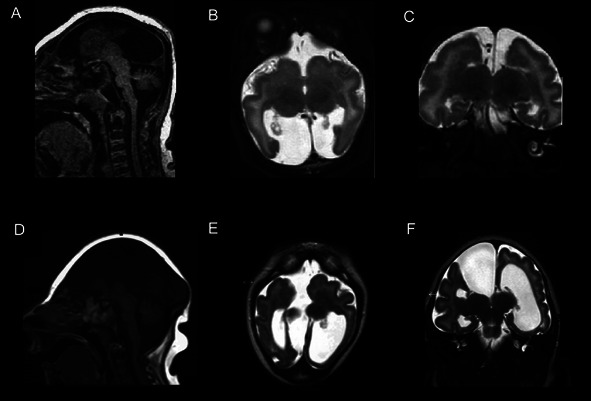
Brain MRI of Patient LP97-141a1 showing agenesis of the corpus callosum on sagittal T1 view (A). There is also increased extra-axial fluid in the posterior fossa. Axial T2 view (B) shows a very simplified gyral pattern, with underdevelopment of the posterior midline cortex at the level of the thalami. There is a polymicrogyria-like cortical dysplasia. Coronal T2 view (C) shows increased extra-axial space in the intrahemispheric fissure with communication with the left lateral ventricle. Brain MRI of Patient LR01-271 demonstrated similar agenesis of the corpus callosum, disproportionately small brainstem, and increased posterior fossa extra-axial fluid on sagittal T1 view (D). Axial T2 view at the thalami demonstrated a large interhemispheric fluid space adjacent to the right lateral ventricle (E). This fluid space is also seen on coronal T2 view (F) and appears to communicate with the right lateral ventricle.
